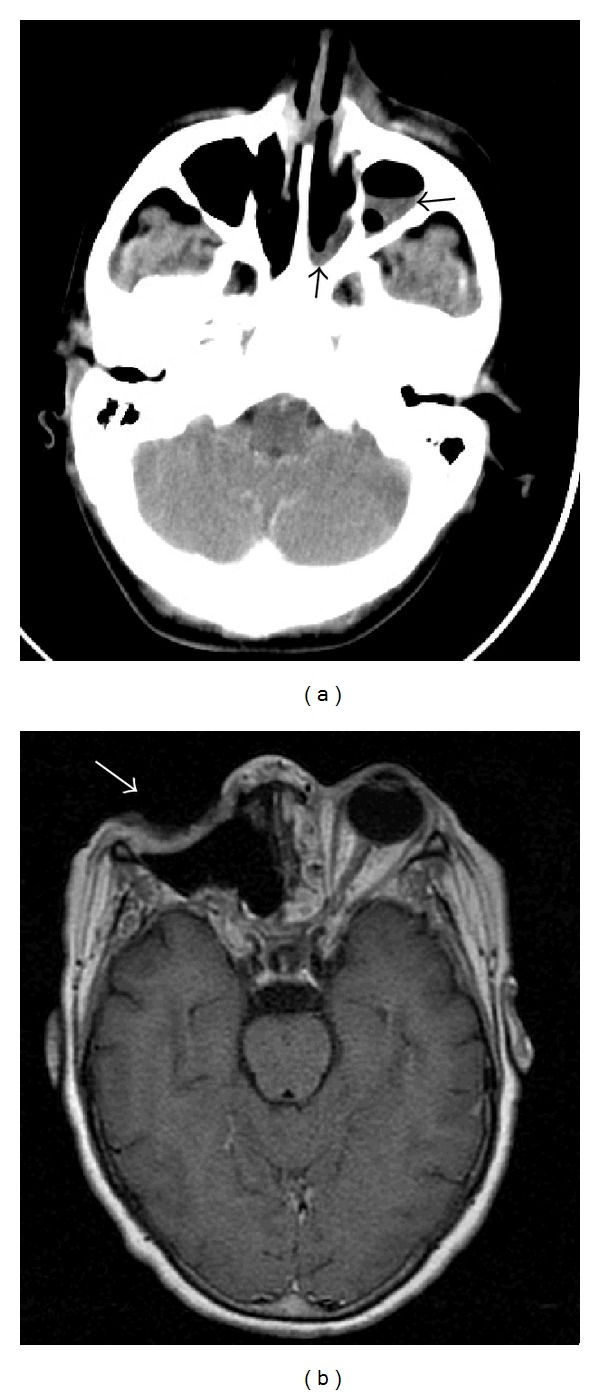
(a) An example of a computed tomography showing sinusitis (black arrows). Biopsies were positive for mucormycosis in this patient. (b) Postsurgical magnetic resonance image (T1 weighted) in a patient with ocular involvement. The patient recovered well.
